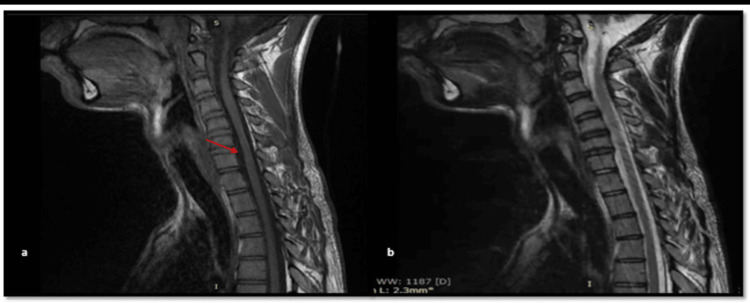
Cervical MRI Sagittal-T1 sequence (a) and sagittal-T2 (b) show cervical spine straightness and moderate atrophy at the C6-C7 level, with enlargement of the subarachnoid space (arrow) without an intramedullary hypersignal.
